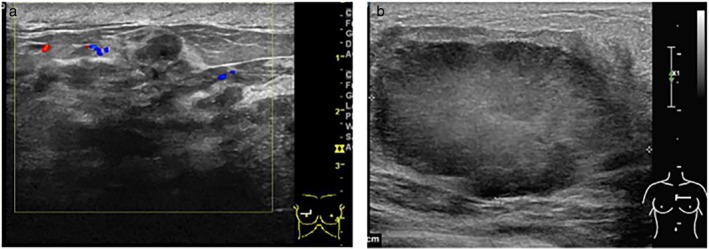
(a) Ultrasound shows the structure of the mammary gland disorder, skin thickening subcutaneous tissue space edema, and low echo area, suggesting breast cancer (Grade III invasive ductal carcinoma). (b) Ultrasound shows a 5.0 × 2.9 × 4.8 cm hypoechoic, undersmooth, irregular, and lobulated mass, which indicates lobular neoplasms (Grade II invasive ductal carcinoma).
